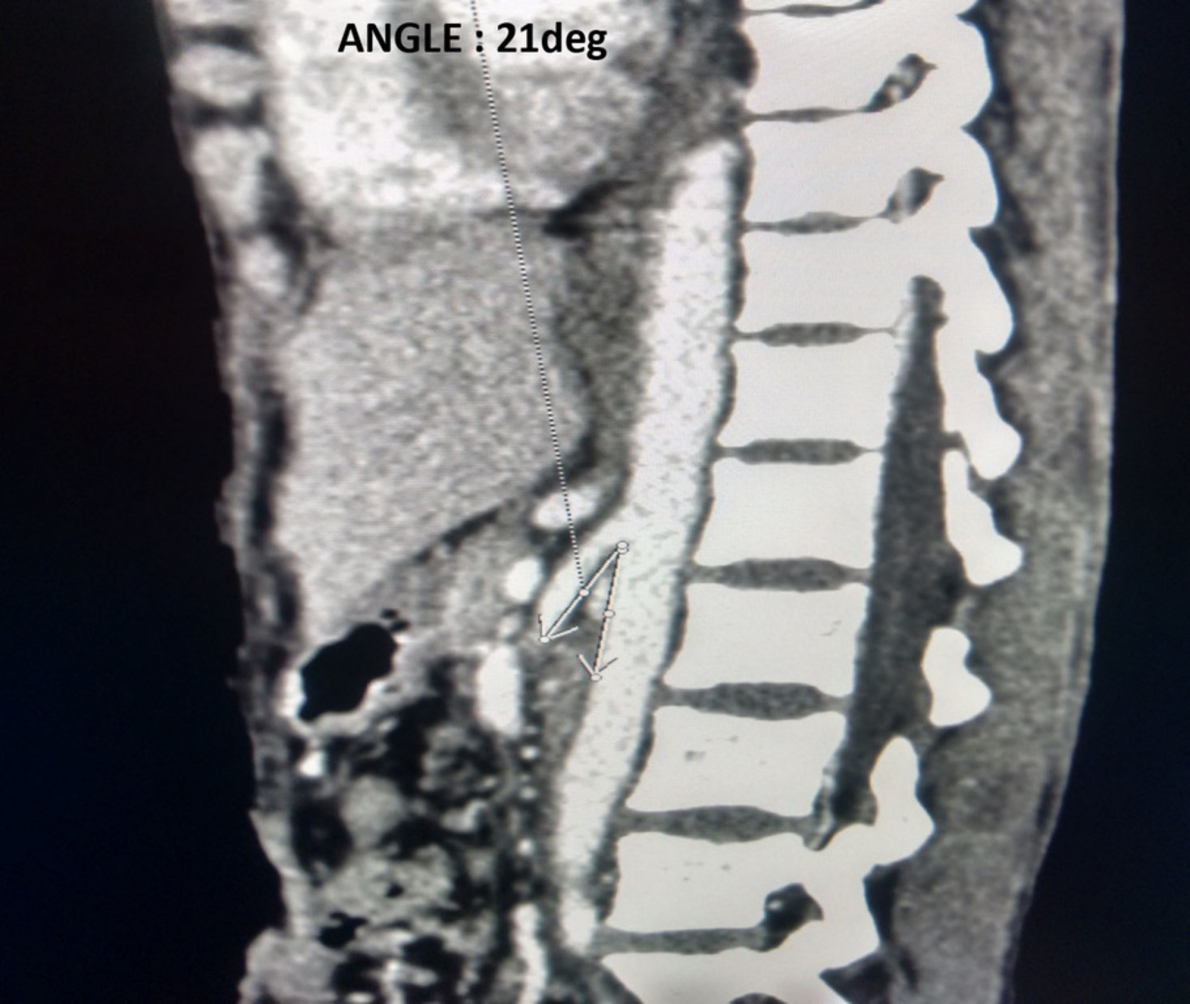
Sagittal section of contrast enhanced computed tomography of abdomen showing increased aortomesenteric angle of 21 degrees (white arrows) after weight gain.
